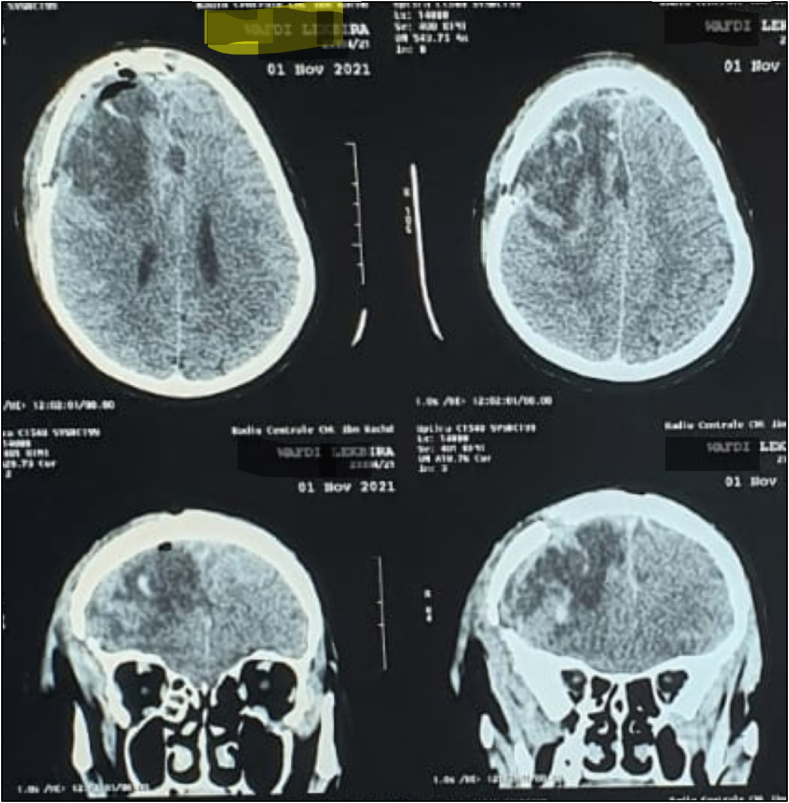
Showing the complete removal of the tumor in control CT scan.
